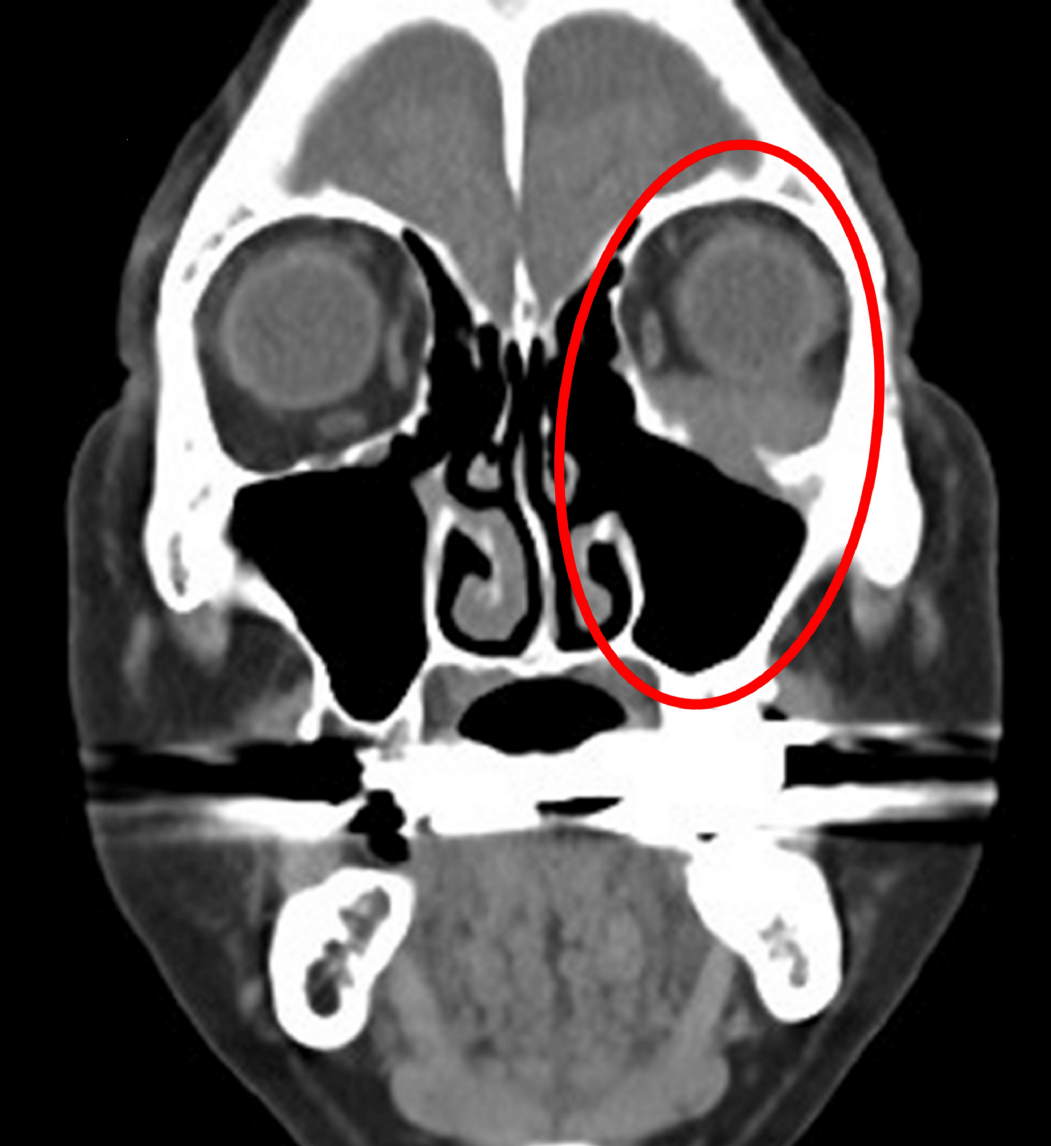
Plain CT image (after six months of treatment) Occupying lesion centered on the left maxillary sinus has improved (circle area). CT, computed tomography
